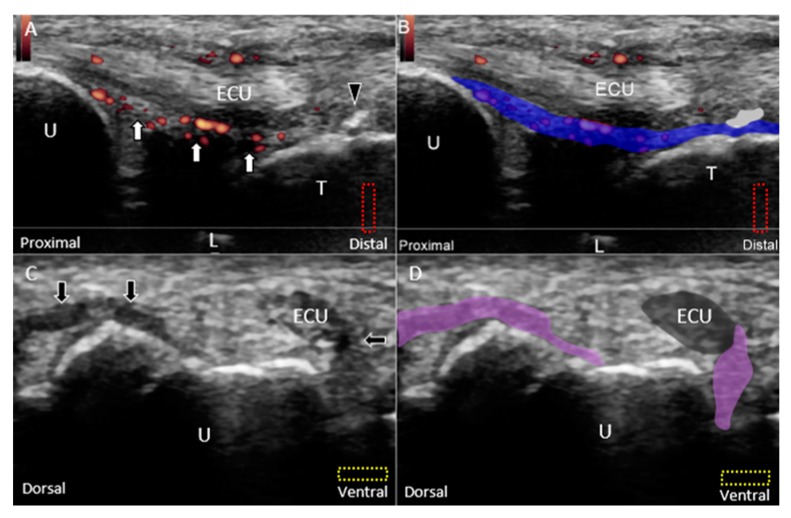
(A) Doppler US and (B) superposed images show the hypervascular and swollen ulnar collateral ligament *(white arrow and blue shade)* and ECU tendon as well as an avulsed fragment of the triquetrum *(black arrowhead and grey shade)* under the long-axis approach. (C) US and (D) superposed US images show rupture of the ECU tendon subsheath *(black arrow and purple shade)* with subluxation of the extensor carpi ulnaris (ECU) tendon *(dark grey shade)* using the short-axis approach. The different color dashed rectangles are in accordance with the transducer positions in Figure 3. US: ultrasound; U: ulna; L: lunate; T: triquetrum; ECU: extensor carpi ulnaris.
